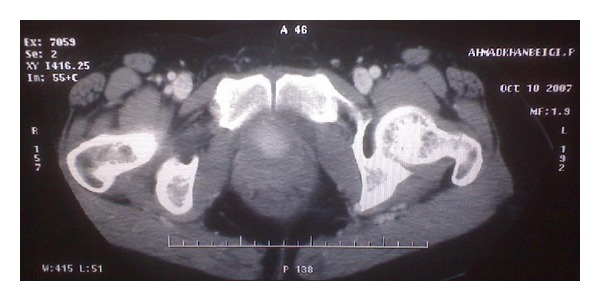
An intravesical mass near bladder neck with hyperdensity in CT scan of patient.
